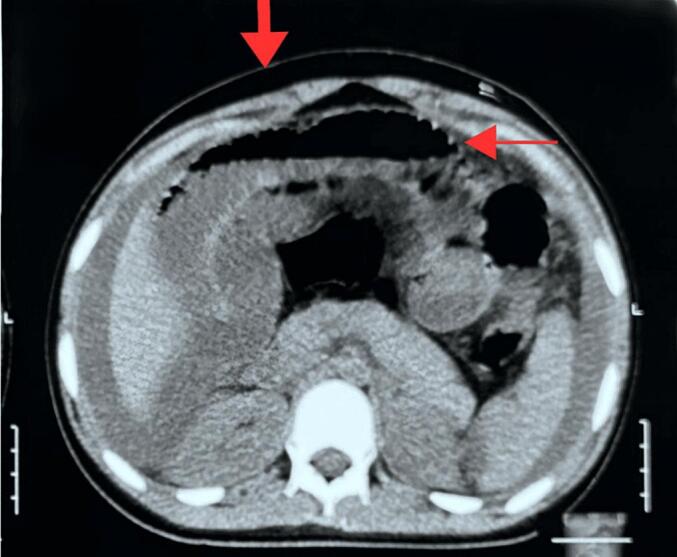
Single slide from contrast-enhanced CT scan, with marked lesions showing ascites and bowel distension.
